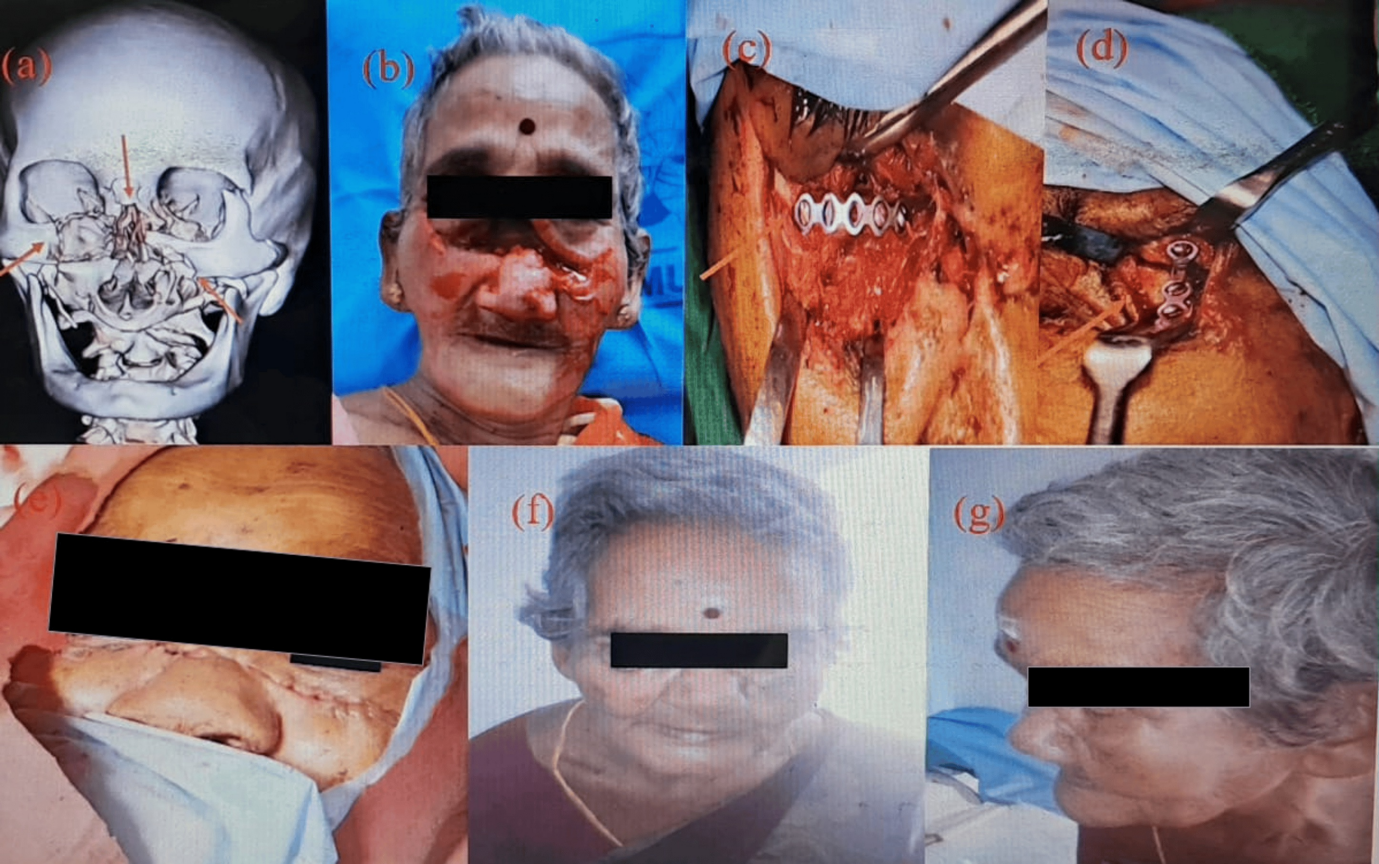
Multiple midfacial fractures a: 3D reconstructed CT facial showing multiple midface fractures (red arrows); b: clinical picture showing deep horizontal laceration; c, d: ORIF under GA (arrow); e: postoperative picture; f, g: one-month follow-up ORIF: open reduction and internal fixation; GA: general anesthesia Written informed consent to include this image in an open-access article was obtained from the patient.
